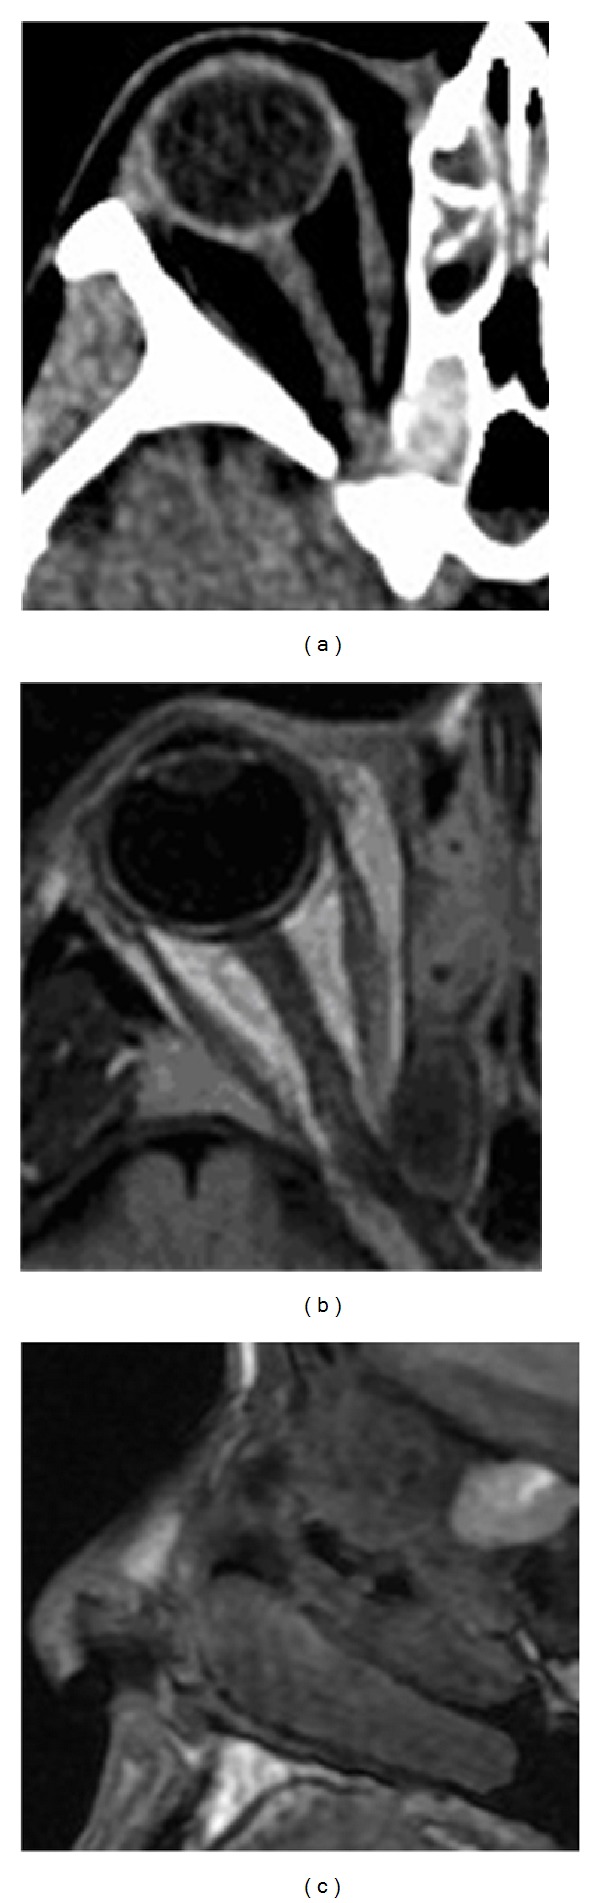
(a) Axial CT showing the mucocele of the right onodi cell. (b) + (c) Fluid attenuated inversion recovery (FLAIR) axial MR image (b) and T2 weighted sagittal MR image. (c) Demonstrating onodi mucocele with close proximity to the optic nerve.
